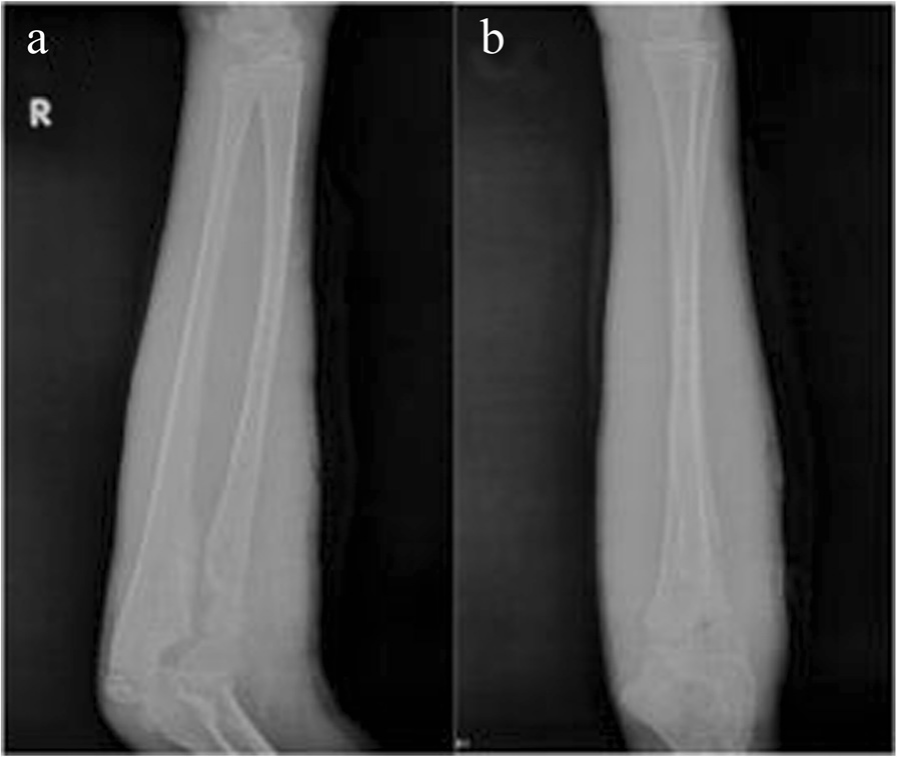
Follow-up radiographs. (a) Oblique and (b) anteroposterior radiographs at 16 months after surgery showed that the anatomical relationship of the humeroradial joint was normal; the osteotomy site was healed well without malunion or ischemic necrosis; and no tumor recurrence was present.
